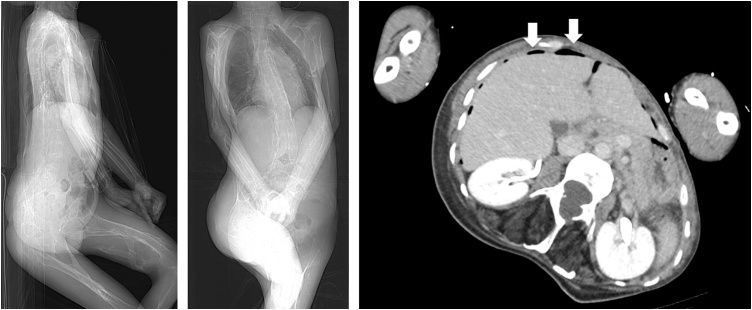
Abdominal CT image prior to surgery showed the presence of intraabdominal free air. The patient’s limbs were hardened and unable to extend.
